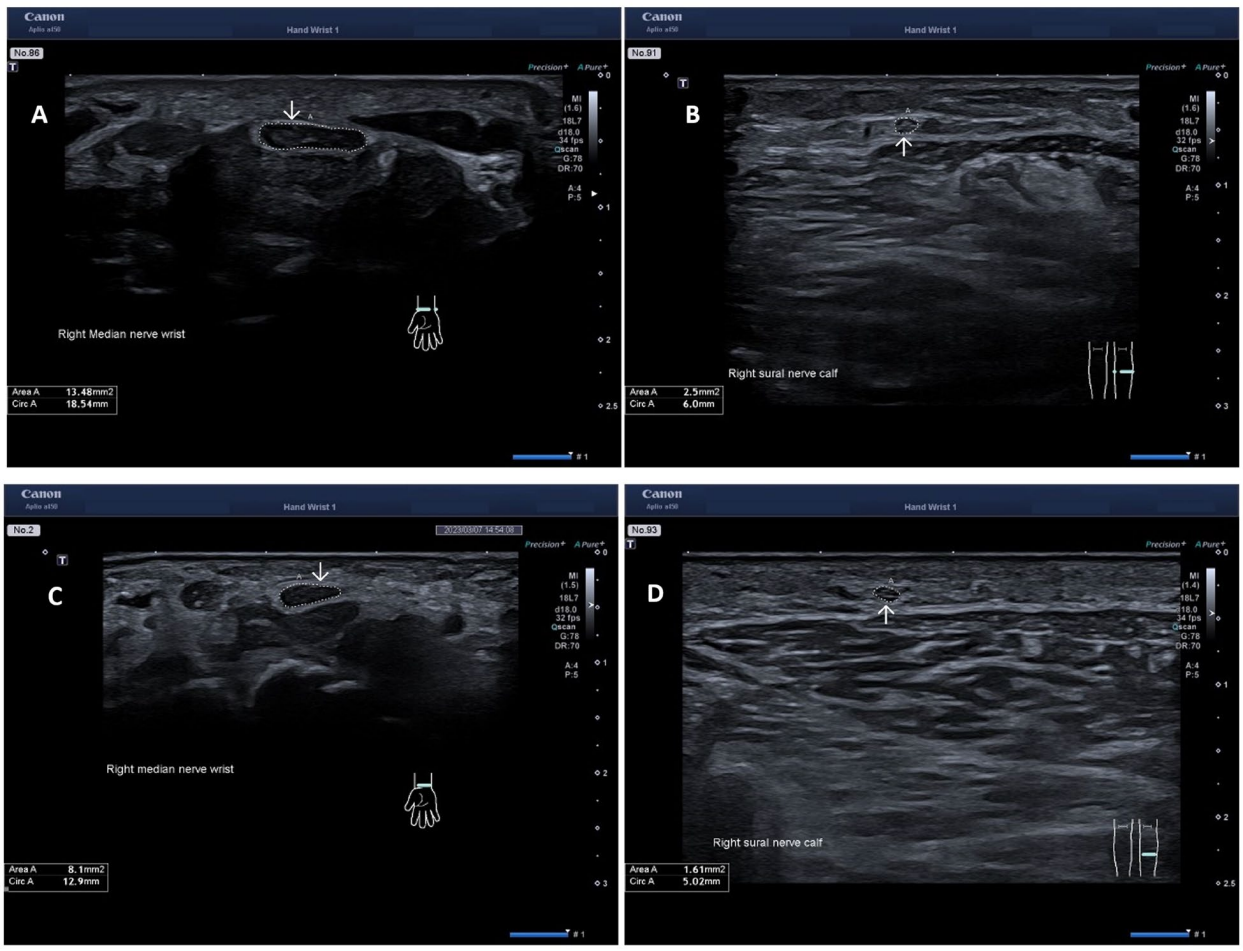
Ultrasound images in a patient with hereditary transthyretin amyloidosis (A) of the median nerve at the wrist, proximal to the carpal tunnel, (B) of the sural nerve, and in a healthy control (C) of the median nerve at the wrist, proximal to the carpal tunnel, (D) of the sural nerve. Arrows showing the cross-section area (CSA) mm^2^.
